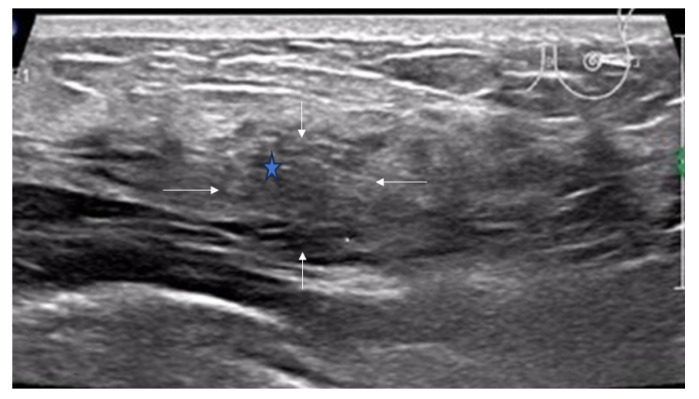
Ultrasound features of non-mass breast lesions of Type V. A case of DCIS. Ultrasound shows no obvious hypoechoic areas (indicated by the white arrows), only scattered hyperechoic foci (indicated by blue five-pointed star).
